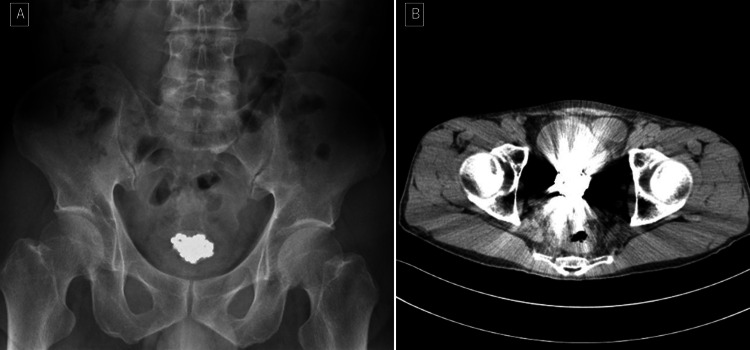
Bladder foreign body on abdominal X-ray examination (A) and on abdominal CT scan (B).
